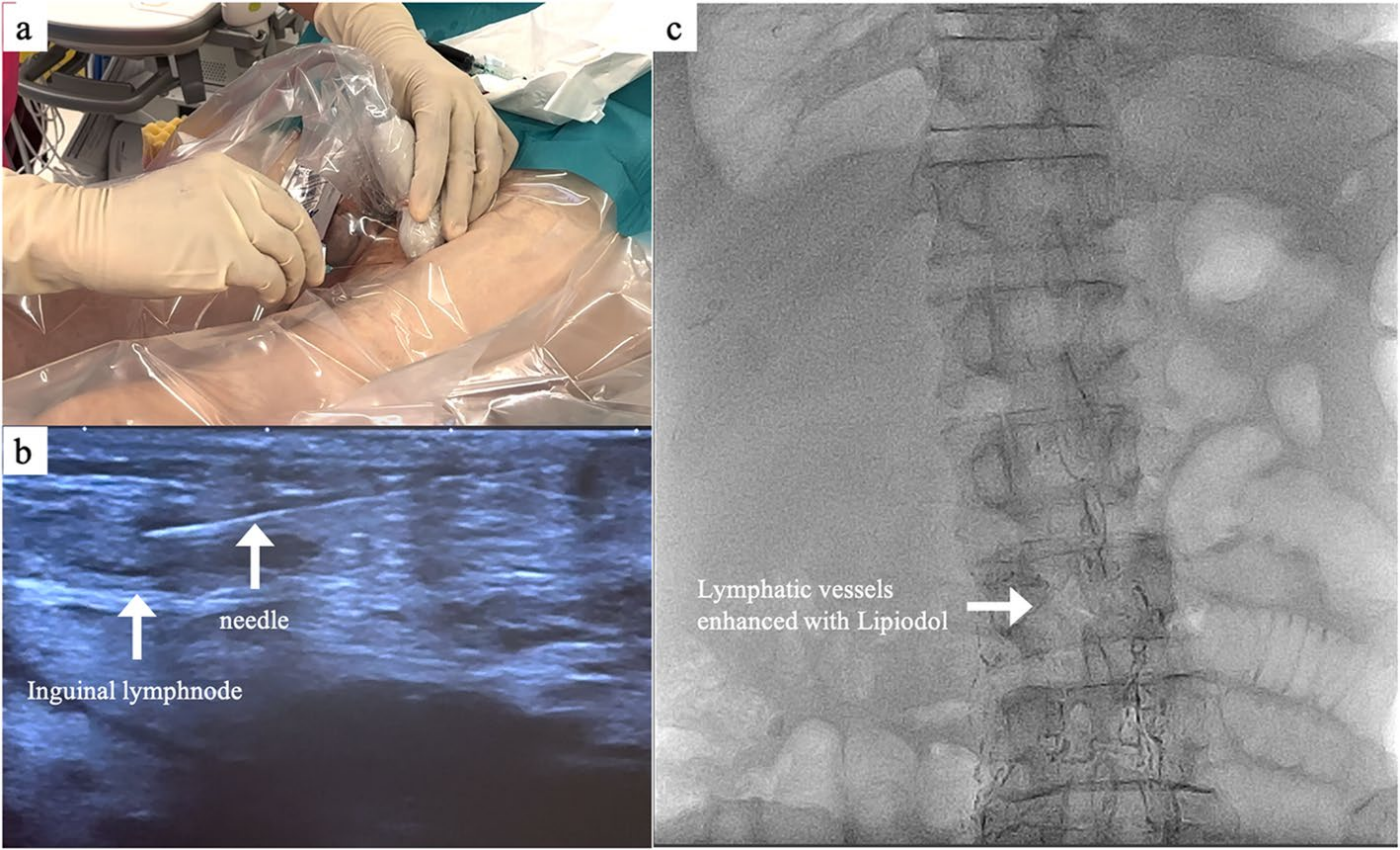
Ultrasound-guided inguinal lymph node administration of indocyanine green. After general anesthesia induction, with the patient in the supine position, an ultrasound-guided left-sided inguinal lymph node injection of Lipiodol was performed. Under fluoroscopy, Lipiodol flow injected into the lymphatic vessels was confirmed
